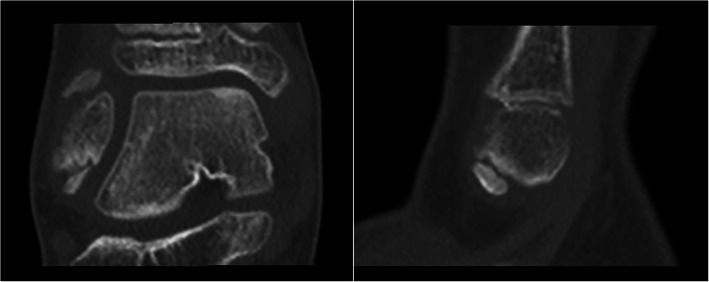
Anteroposterior and lateral CT scan. The non united fragment is visible and classified as a type 7b in the Ogden classification
